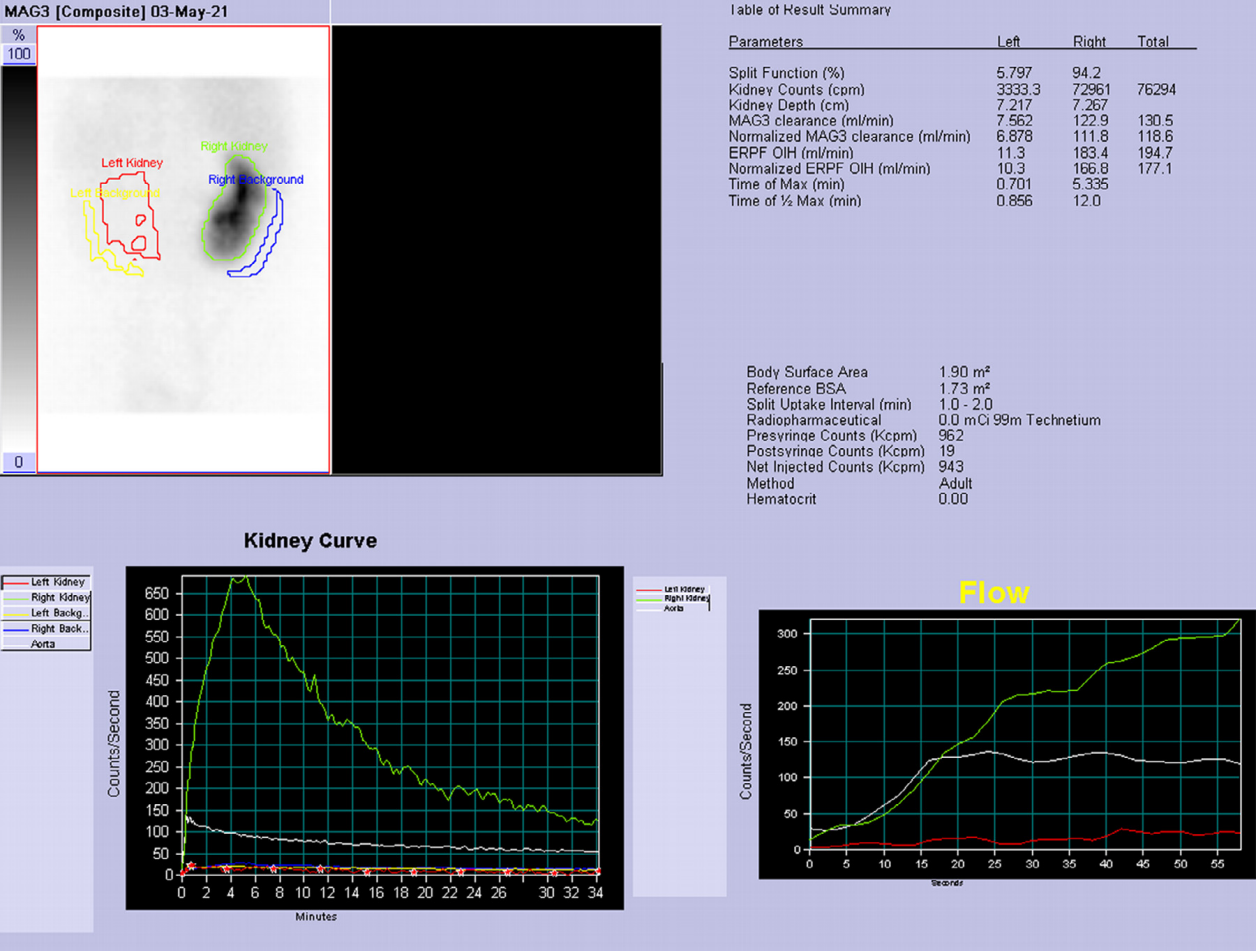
Renal scintigraphy. Nonfunctional left kidney.
